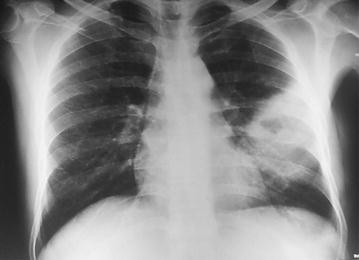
Chest x-ray posterior–anterior view on admission (04/09/2014) showing homogenous opacity with a cavitary lesion occupying parts of left middle and lower zones
